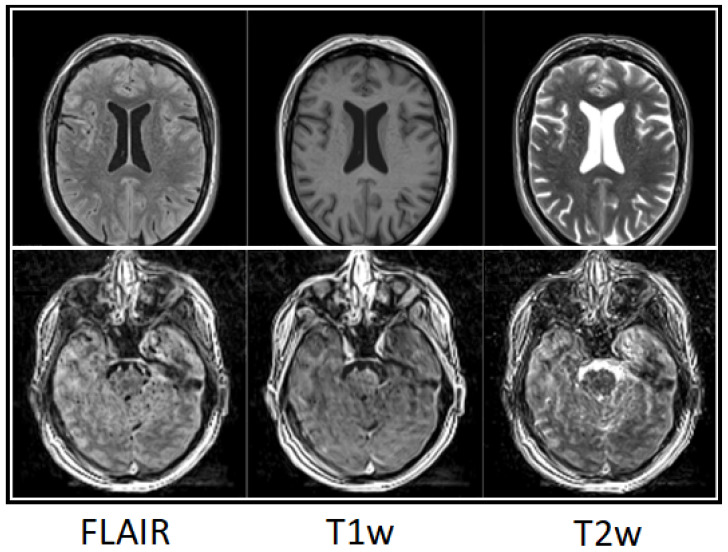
Three-dimensional SI examples of perceived motion on derived image weightings (from left, FLAIR, T1w, T2w) rated on T1-weighted 3D SI. Patient images in upper row ratings (cMRI) R1, R2, R3: 92, 95, 92, and lower row 11, 18, 29, respectively.
